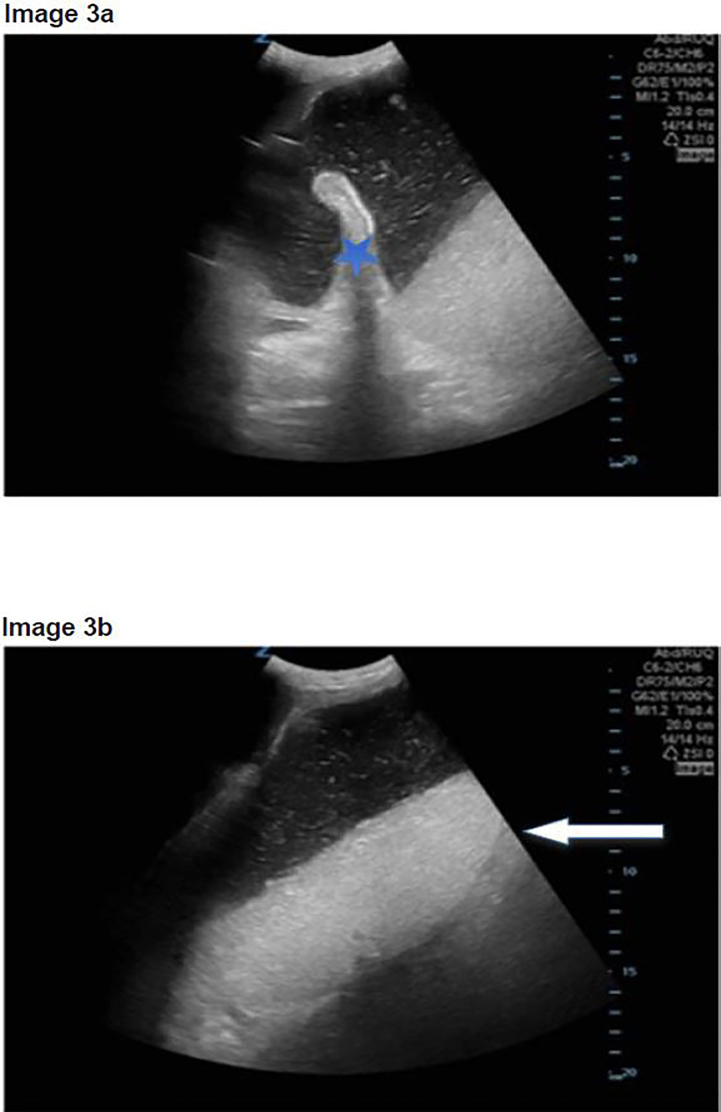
a) Point-of-care ultrasound (POCUS) with dilated stomach with visible stomach fold (blue star) and bidirectional flow of stomach contents; b) POCUS with “black-and-white cookie” sign seen by white arrow with division of stomach contents.
